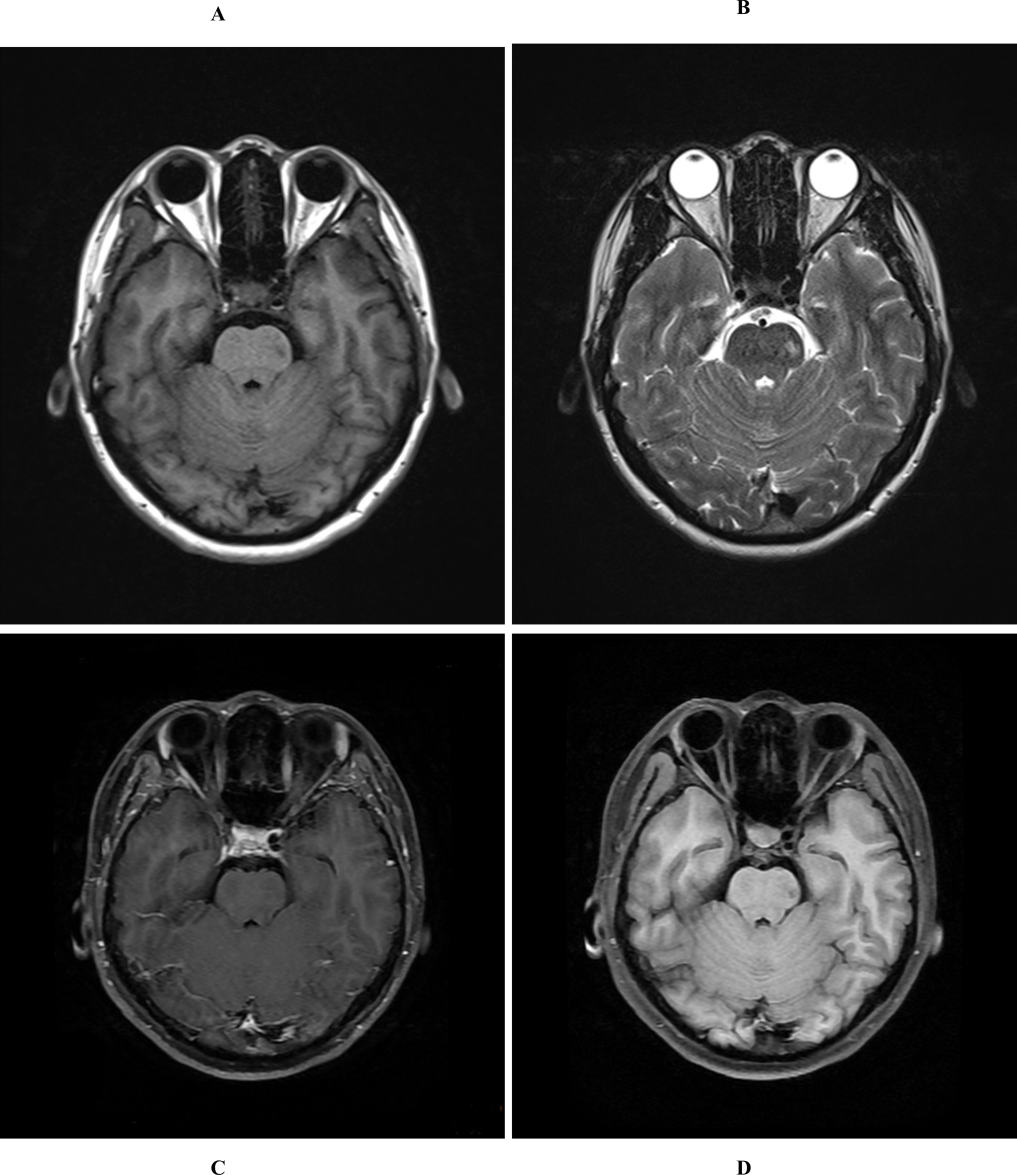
Cranial MRI of the patient. (A) T1-weighted imaging; (B) T2-weighted imaging; (C) The contrast-enhanced cranial MRI; (D) Fluid-attenuated inversion recovery (FLAIR) imaging.
